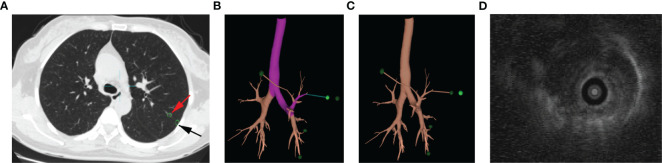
ENB-guided MWA procedure: targeting nodule in the chest CT (A), red arrow points to the GGO, and black arrow points to the subpleural point of the GGO; planning of targeting GGO (B); subpleural point added along the airway route (C); EBUS-GS image of nodule (D).
